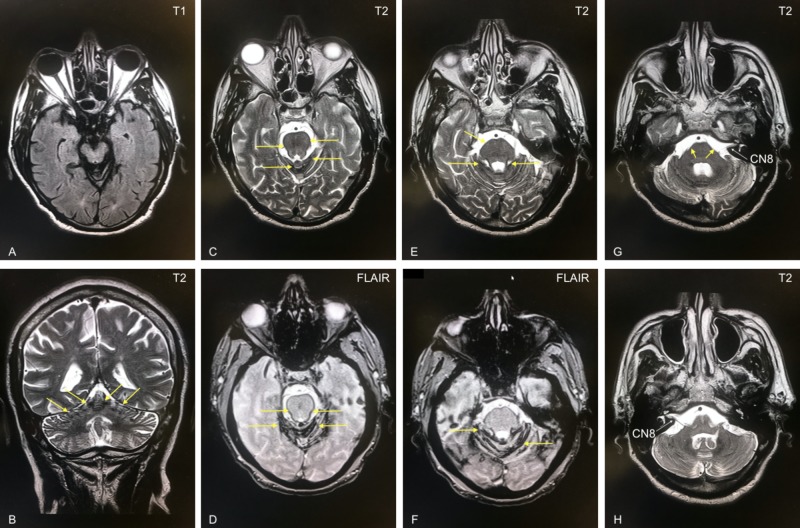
Brain MRI Axial plane multisequence T1, T2, and FLAIR MRI showing the midbrain (A), pons (C-F), and cerebellum (G, H). Coronal plane T2-weighted MRI (B). Hemosiderin hypointensities (black) noted along the cerebellar folia and basal cisterns in both coronal and axial planes (yellow arrows). Cranial nerve VIII (CN8) noted (white arrows). FLAIR, fluid-attenuated inversion recovery
